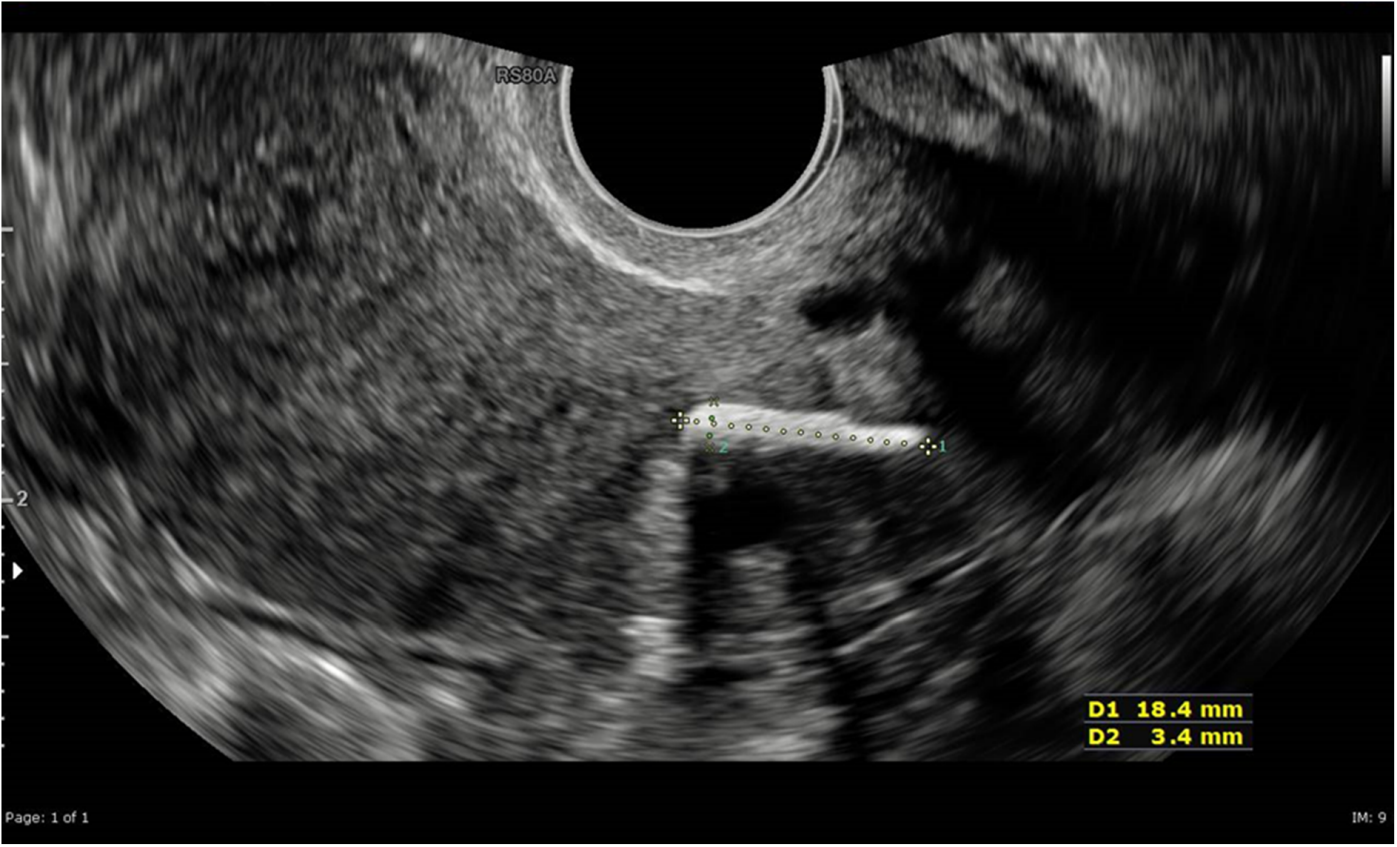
Transvaginal ultrasound showing possible foreign body
